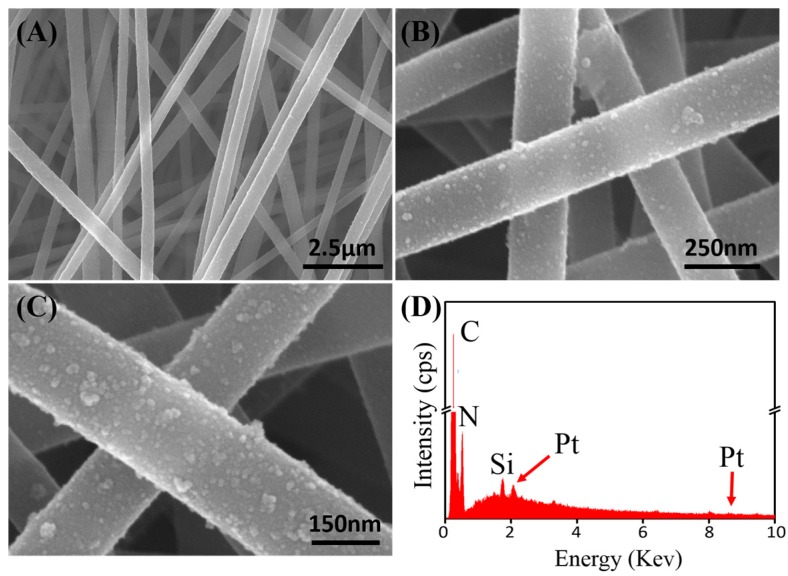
(A) SEM image of electrospun PAN/APS nanofibers; (B,C) SEM images of PAN–PtNPs hybrid nanofibers with different magnifications; (D) Corresponding energy dispersive X-ray detector (EDX) analysis.
